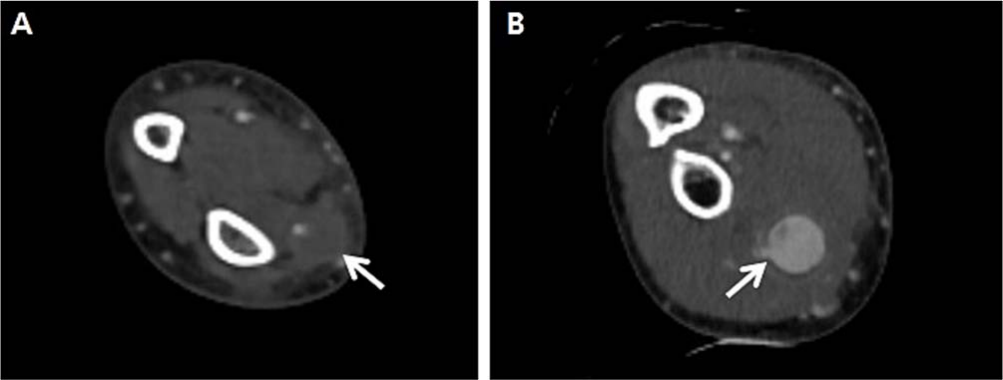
Preprocedural computed tomography of the right upper extremity. (A) Pseudoaneurysm at the distal radial artery which was arterial catheterization site (arrow); (B) Pseudoaneurysm at the proximal radial artery which was not related to arterial catheterization site (arrow).
